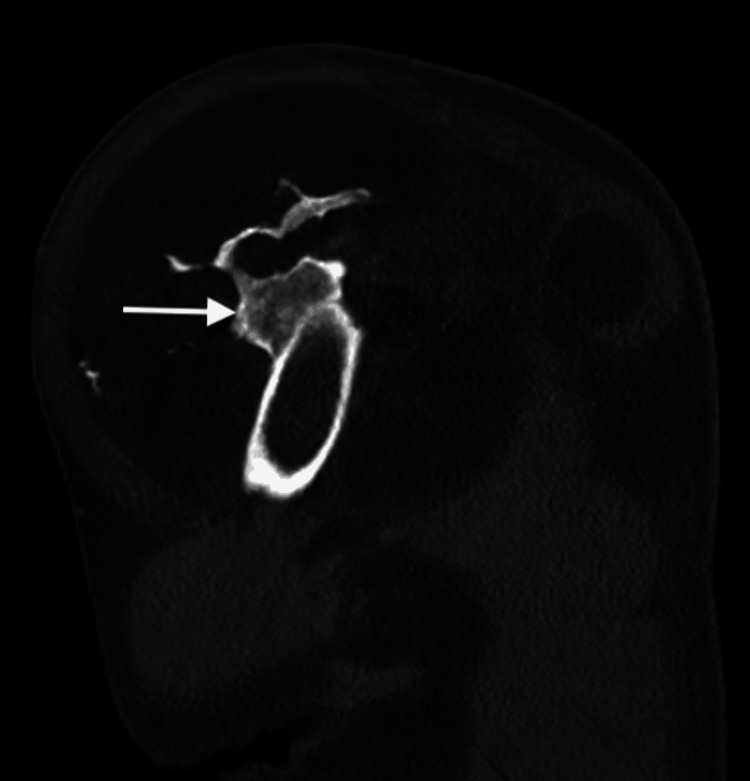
Sagittal bone window CT. A large lipomatous lesion adjacent to the left humerus is visible. An irregular osseous excrescence (arrow) is noted, lacking continuity with the humeral bone marrow.
